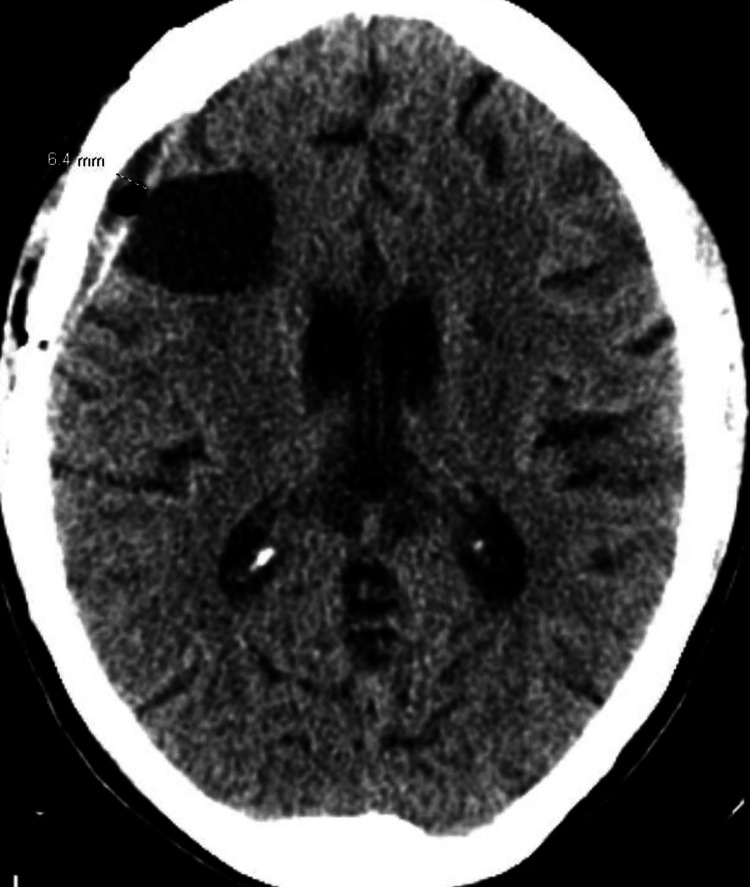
Post-craniotomy head CT without contrast Postoperative changes are evident, with an interval decrease in vasogenic edema around the resection cavity and a residual 6.4 mm fluid collection.
